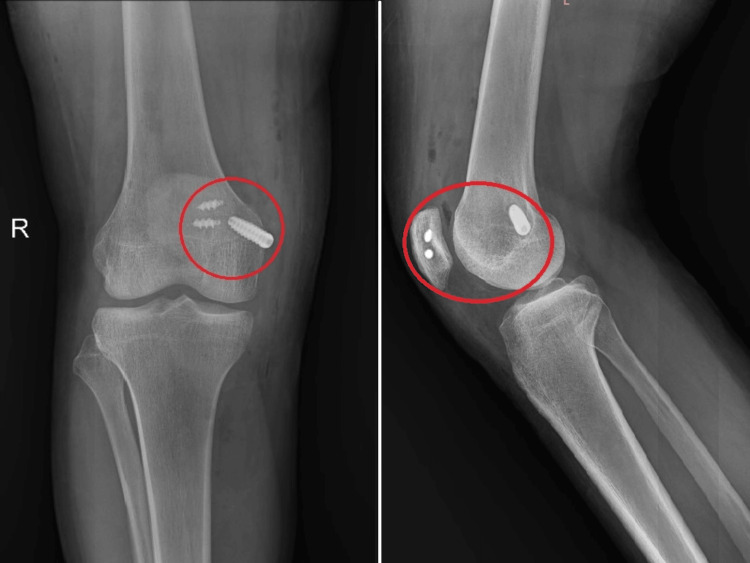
Post-operative X-ray showing patellar suture anchors and interference screw at the Schöttle point of the femur Schottle's point serves as a radiographic reference indicating the isometric point for femoral insertion in the context of MPFL reconstruction. MPFL, medial patellofemoral ligament
